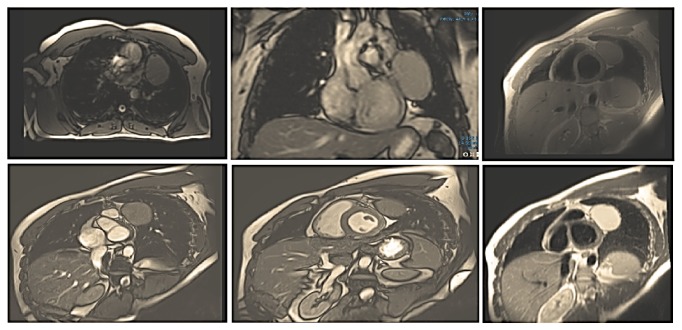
Cardiac MRI showing a para cardiac cyst next to the anterolateral wall of the left ventricle in close contact with the pericardium but with a cleavage plane (Patient 2).
